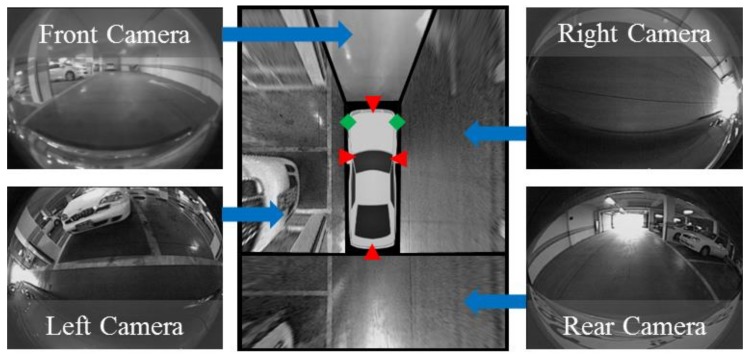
Sensor configuration. Red triangles and green diamonds are four cameras of the AVM system and two ultrasonic sensors, respectively.
